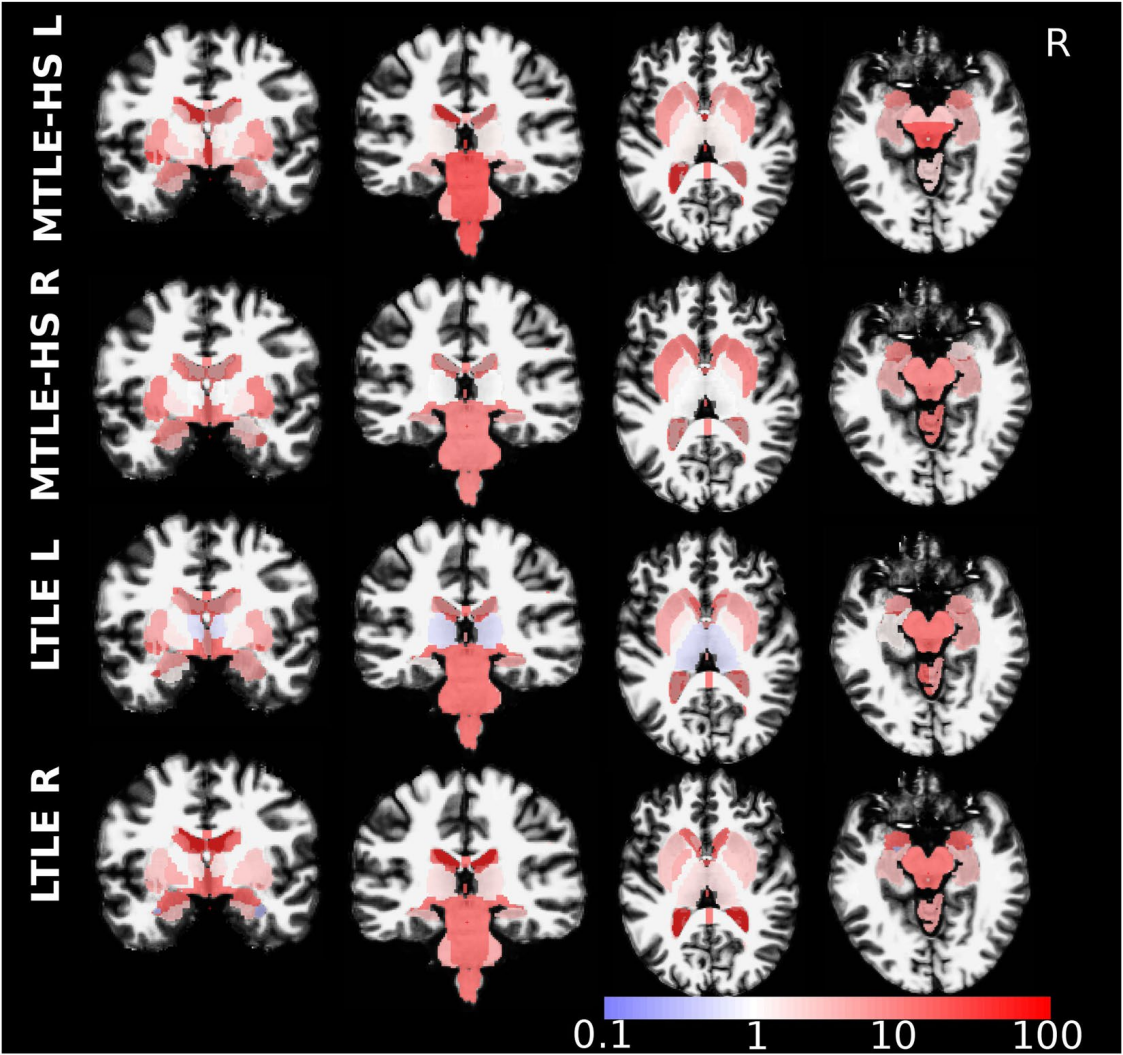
Diagnostic odds ratio of morphometric detections in volume segmentations with respect to expert MRI assessment. Coronal and axial slices are in neurological orientation, i.e. the left hemisphere appears on the left.
